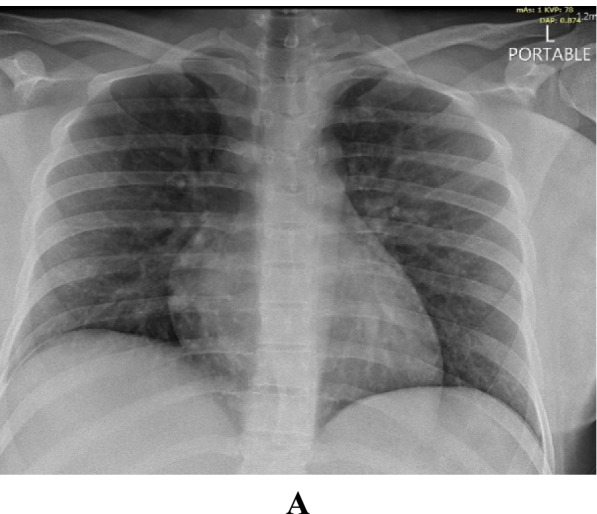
A Patient 2 - Chest X-ray on admission
